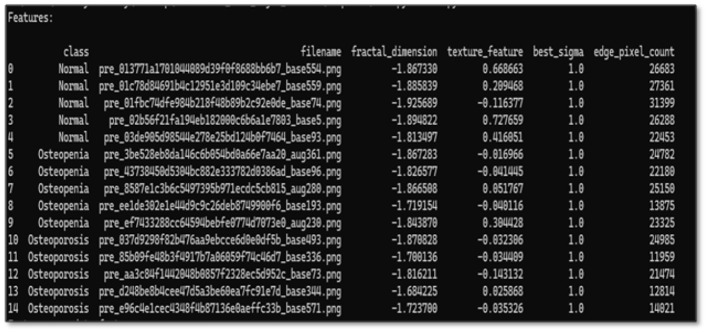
Combined feature extraction results for all images using Multi-Class Knee Osteoporosis X-ray dataset.
